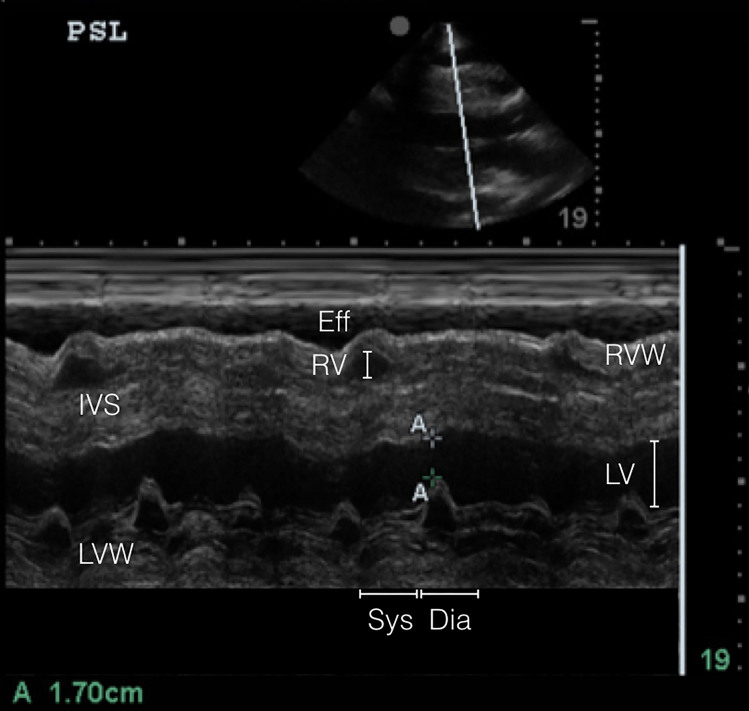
Abnormal point-of-care transthoracic echocardiogram (parasternal long axis in M-mode) - right ventricular wall collapse as the mitral valve begins to open in early diastole. *Eff*, effusion; *RV*, right ventricle; *RVW*, right ventricular wall; *IVS*, intraventricular septum; *LV*, left ventricle; *LVW*, left ventricular wall; *Sys*, systole; *Dia*, diastole.
